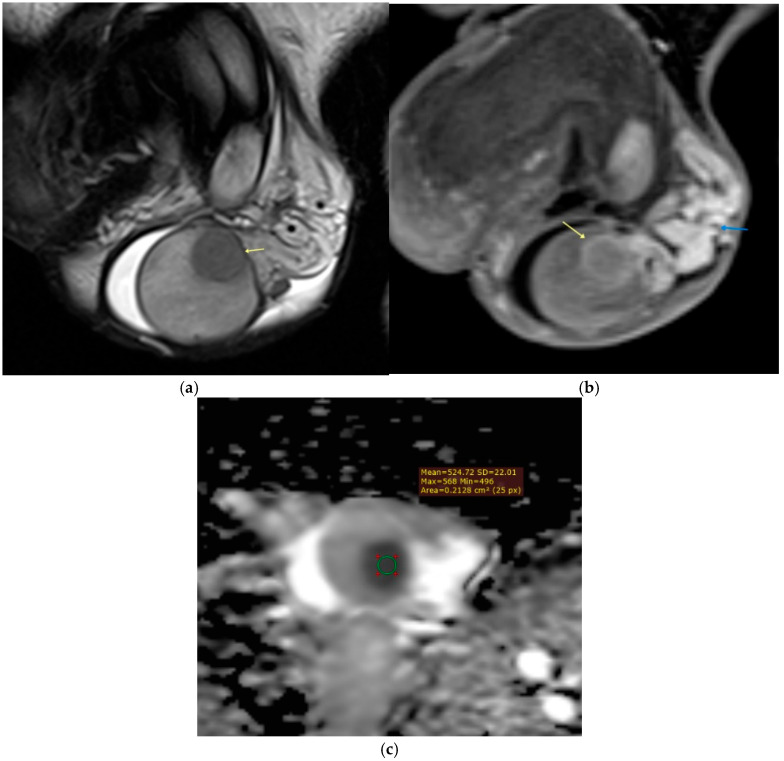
Seminoma of the left testis. (a) Coronal T1-weighted image depicts a well-circumscribed, homogeneous, hypointense intratesticular mass (yellow arrow). (b) Coronal post-contrast T1-weighted image shows homogeneous enhancement of the lesion; note the dilated, tortuous pampiniform veins consistent with varicocele (blue arrow). (c) Corresponding ADC map demonstrates pronounced diffusion restriction within the tumor, with an ADC value of approximately 0.5 × 10^−3^ mm^2^/s.
